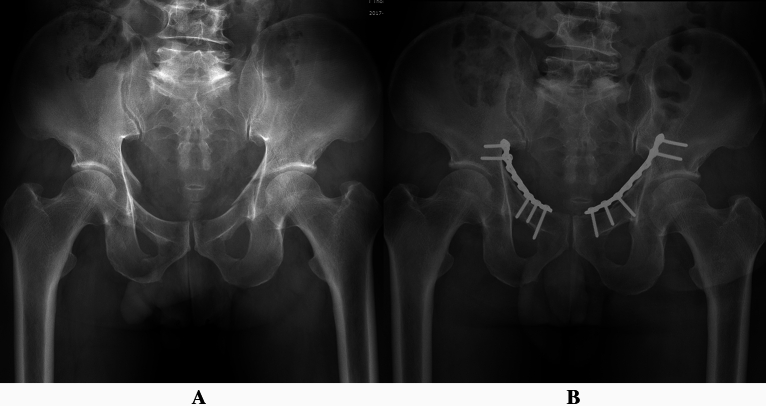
Preliminary clinical application of the technique proposed in this study. (A) Preoperative anteroposterior radiograph of a male patient with bilateral acetabular fractures; (B) extra-articular screw placement was accomplished based on the results obtained in this study.
